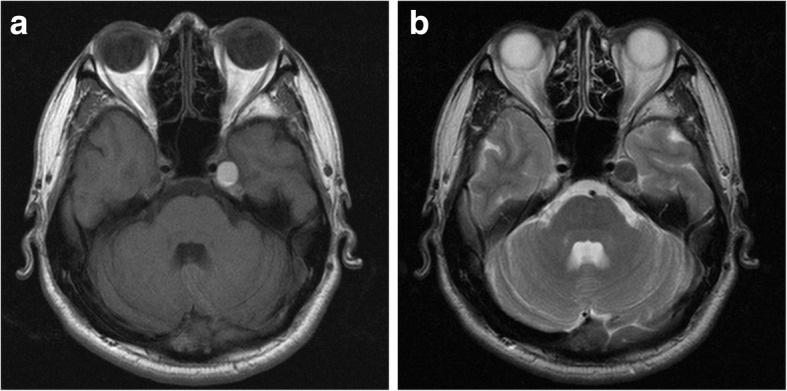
Magnetic resonance imaging demonstrating a tumor 10 mm in diameter. a T1-weighted axial images (repetition time 667/echo time 9) revealed a homogeneously high signal tumor in Meckel’s cave. b T2-weighted axial images (repetition time 5200/echo time 98) revealed a low signal tumor exerting pressure on trigeminal nerve
